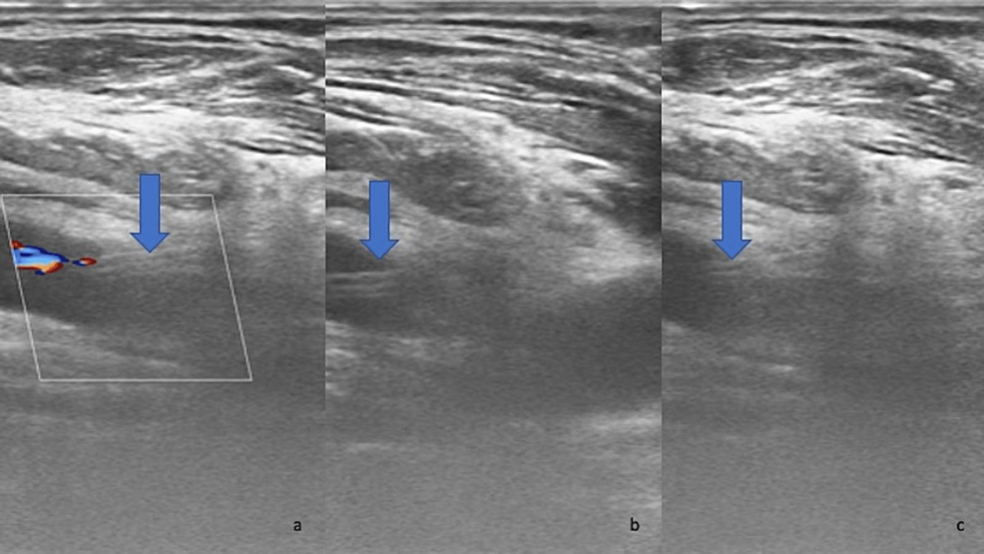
Duplex ultrasound identifying the misplaced catheter
